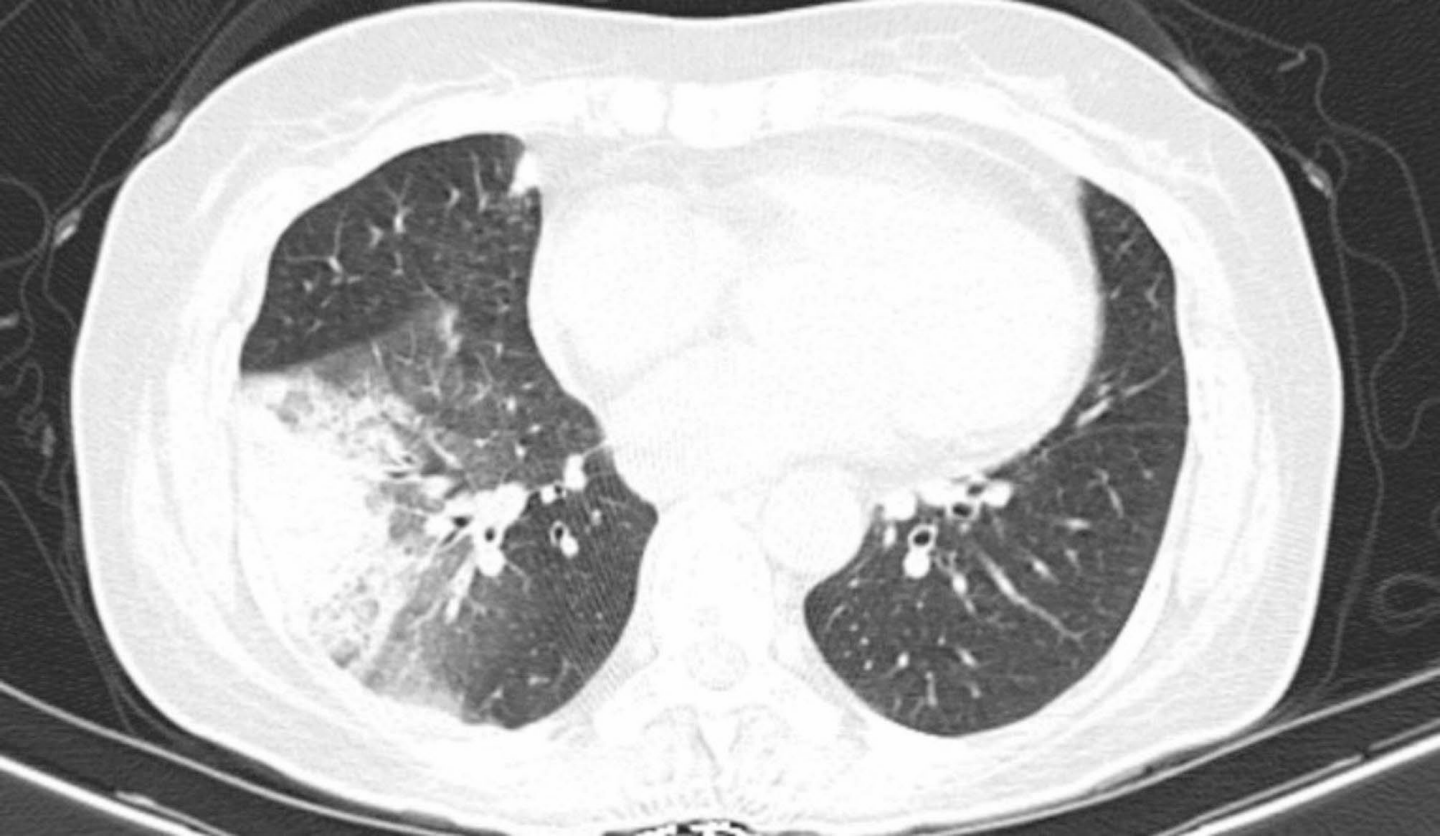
Lung CT scan shows consolidation and opacity of the Grand Glass of the upper segment and posterior basal of the Right lower lobe. Mild plural effusion is seen
